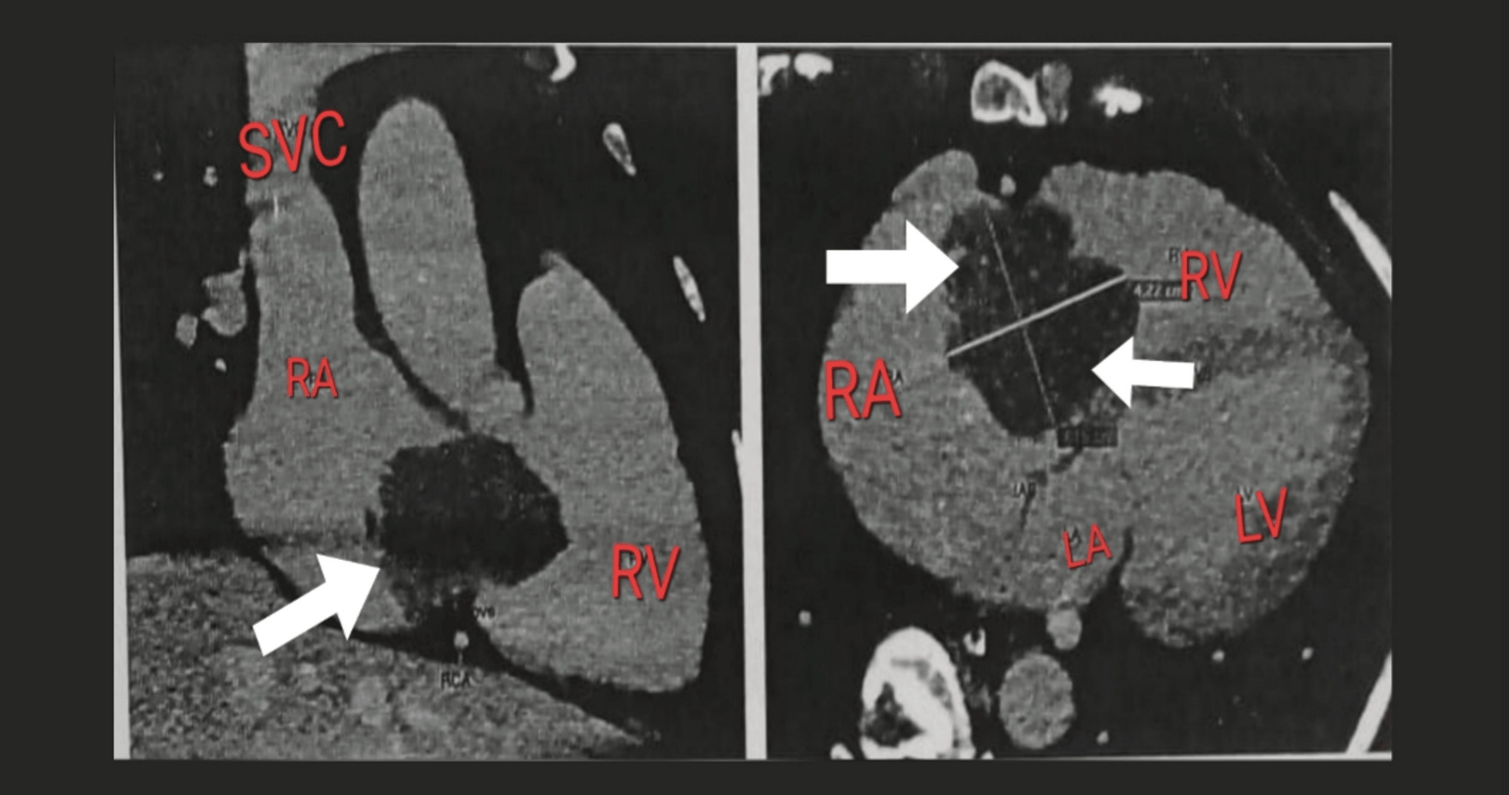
CT cardiac angiogram. The arrow shows the right atrial mass. The figure shows the dilated right atrium measuring 3.3 × 4.2 × 4.5 cm. RA = right atrium; LA = left atrium; RV = right ventricle; LV = left ventricle; SVC = superior vena cava
